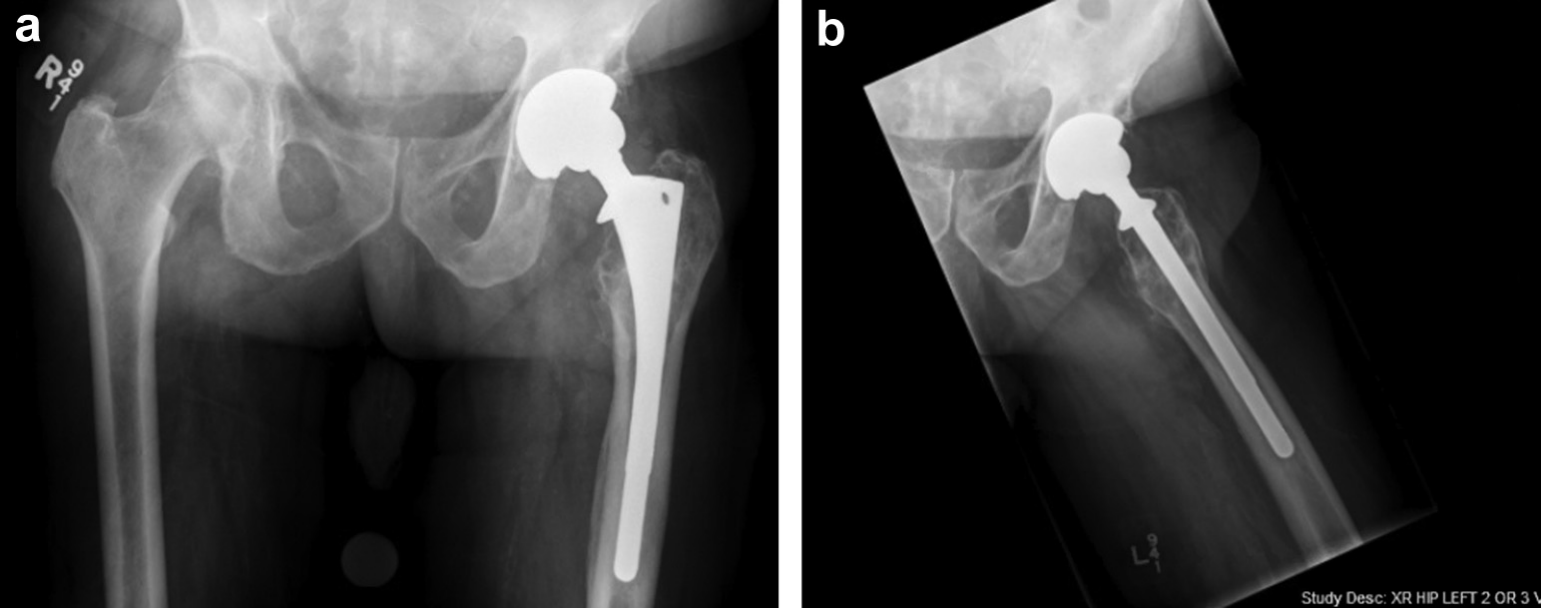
Antero-posterior (a) and frog lateral (b) 1-year follow-up radiographs, demonstrating a stable appearance and no new osteolysis or signs of loosening.
